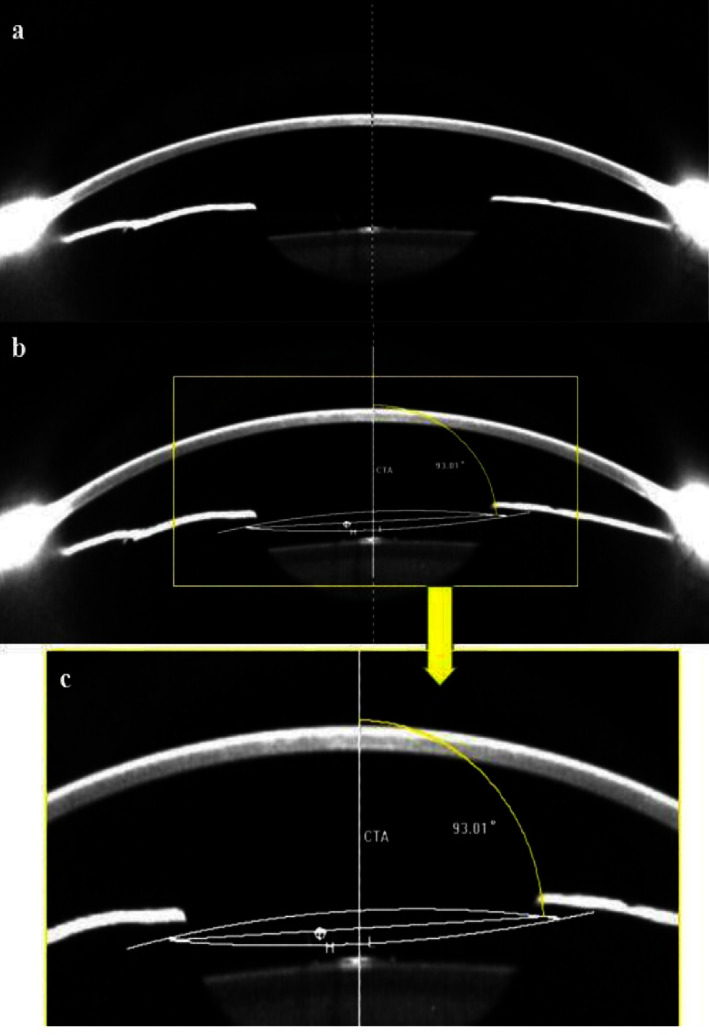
The anterior segment pentacam image with the ICL horizontal. (a) The white point *H* on the processed image (b) indicates the ICL central hole, while line *L* represents the plane of the ICL; the ICL axis runs perpendicular to the ICL plane. (c) The ICL tilt degree (between the CTA and the ICL axis) is 93.01°–90° = 3.01°.
